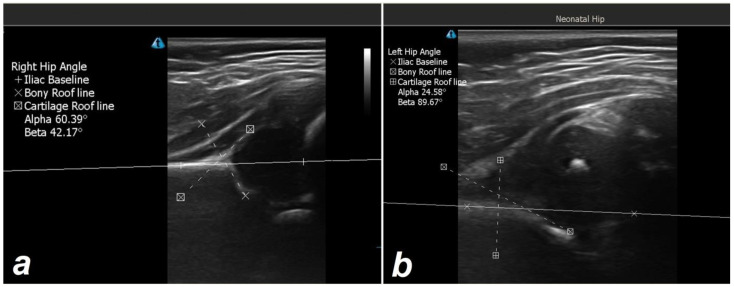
Ultrasonic examination of a patient without (a) and with developmental hip dysplasia (b).
